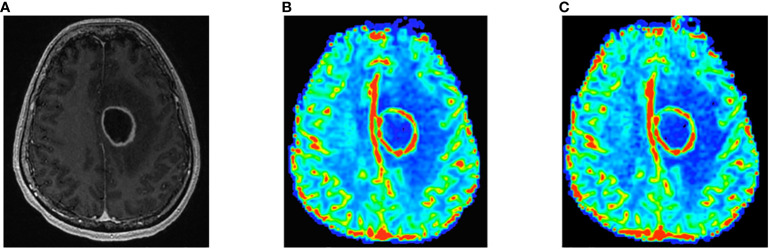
Contrast-enhanced T1-weighted image shows left frontal rim-enhancing mass consistent with GBM (A). Note similarity of 60°/P+/C+ (B) and 30°/P-/C+ (C) color srCBV maps.
